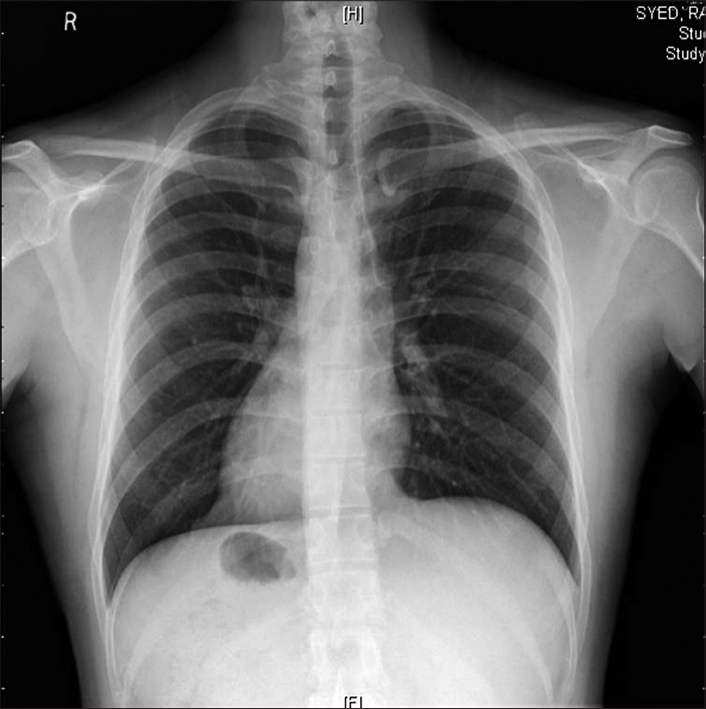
X-ray chest showing dextrocardia and gastric air bubble on right side i.e. situs inversus totalis.
